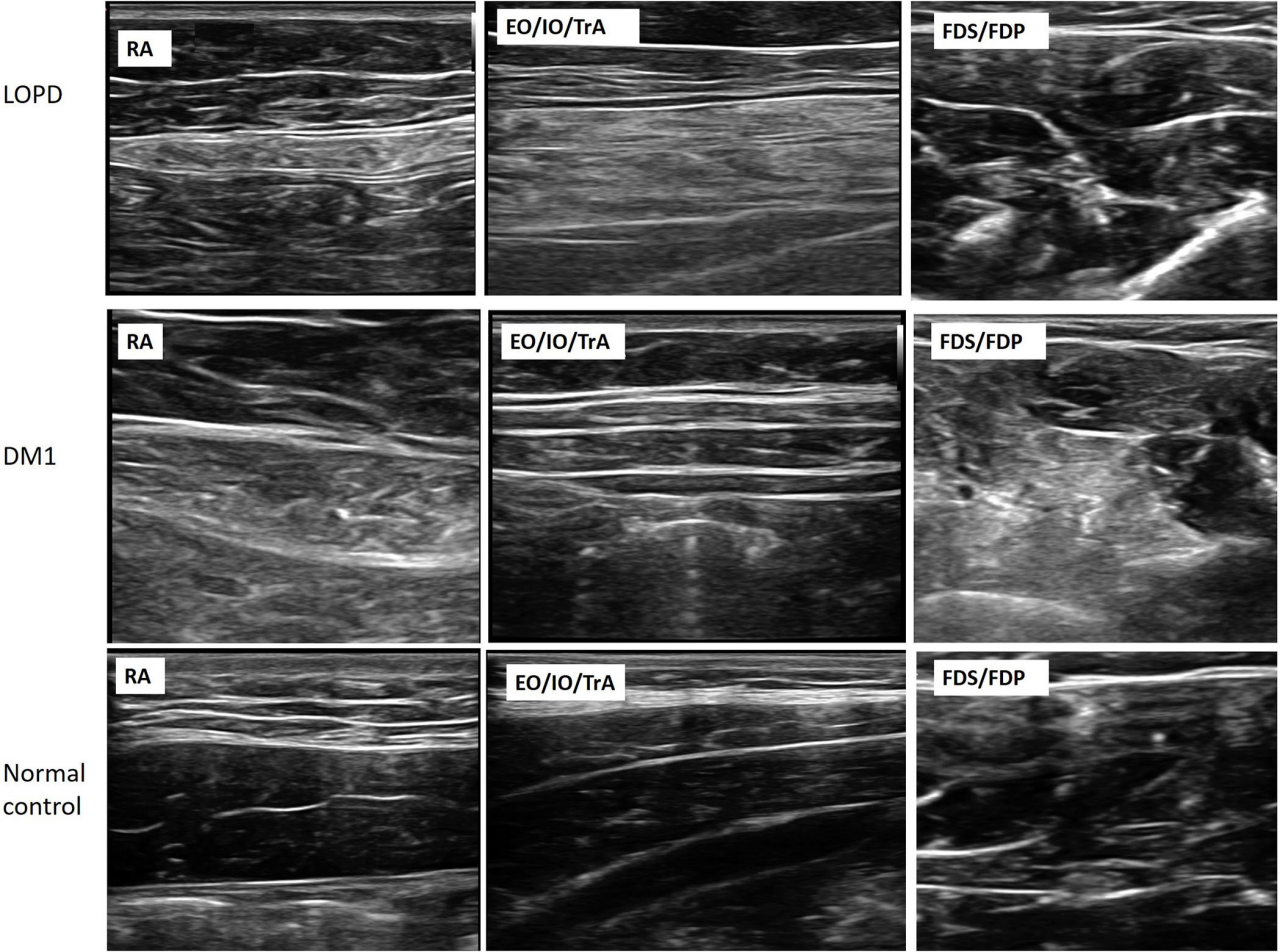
Muscle ultrasonography of RA, EO/IO/TrA, and FDS/FDP muscles in the patients with LOPD, DM1, and normal controls. The images demonstrate increasing muscle echogenicity and atrophy of trunk muscles and normal echogenicity of FDS/FDP muscles in the LOPD group. For the DM1 group, the images show mildly increasing echogenicity in trunk muscles and increasing echogenicity in FDS/FDP muscles. LOPD, Late-onset Pompe disease; DM1, Myotonic dystrophy type 1; HC, Healthy control; RA, Rectus abdominis; EO, External oblique; IO, Internal oblique; TrA, Transversus abdominis; FDS/FDP, flexor digitorum superficialis/flexor digitorum profundus.
